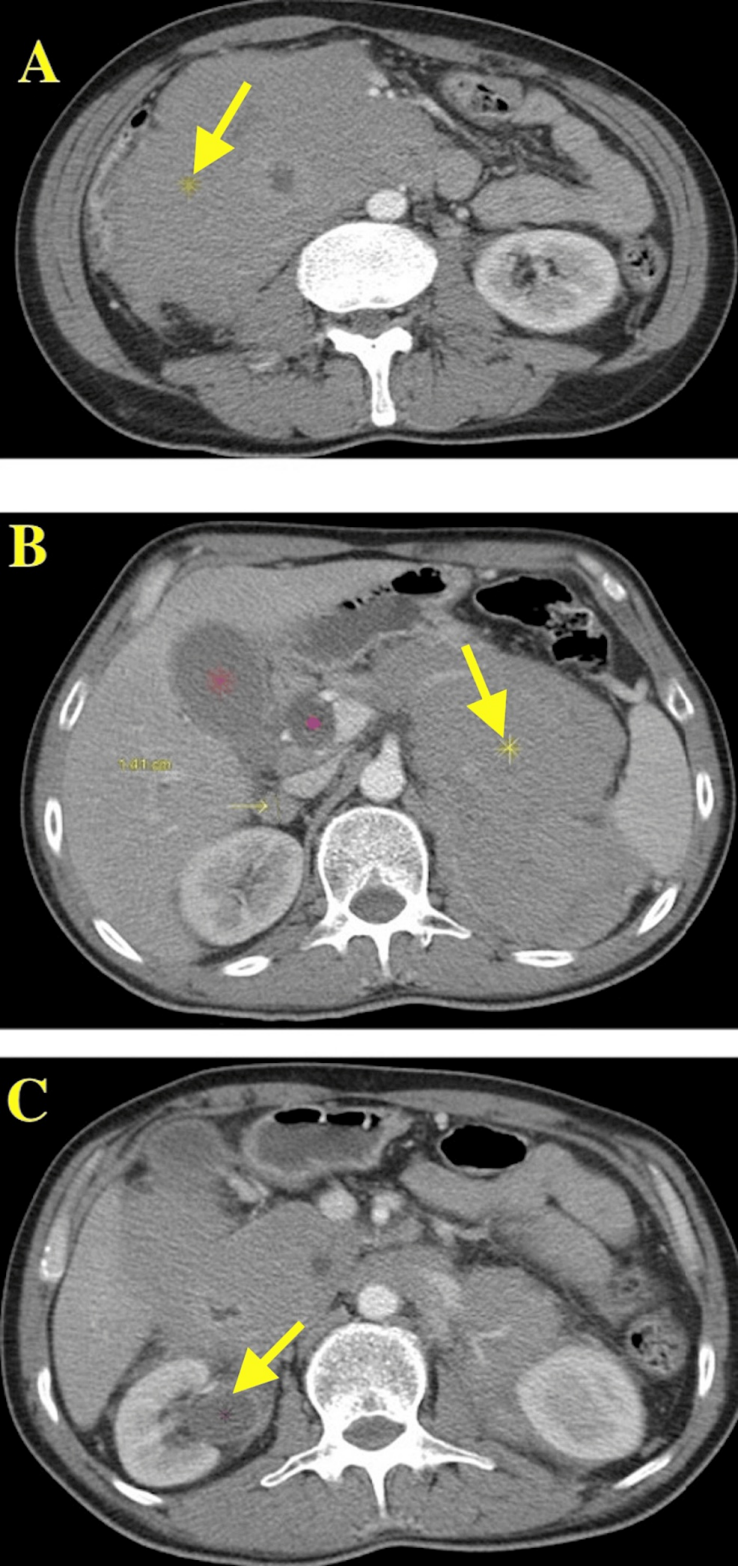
CT abdomen and pelvis. Panel A shows a large right upper quadrant mass. Panel B shows a left upper quadrant mass with gallbladder distension, minimal wall thickening, and some pericholecystic fluid. Panel C: right renal nodule measuring 1.4 cm with right-sided hydroureter most likely due to extrinsic compression from the mass. CT, computed tomography
